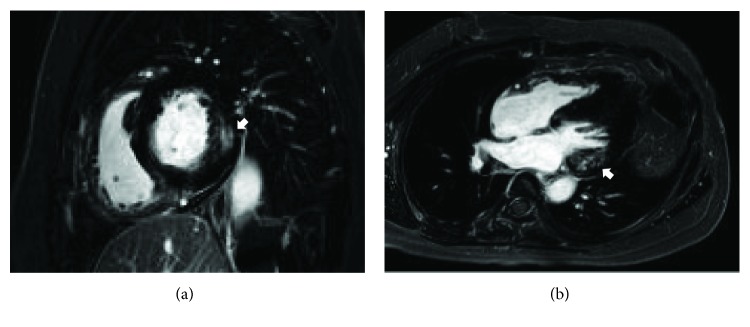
Magnetic resonance imaging. Magnetic resonance imaging showed delayed enhancement (arrows) at the basal segment of LV lateral wall. (a) Short axis view. (b) 4-chamber view.
